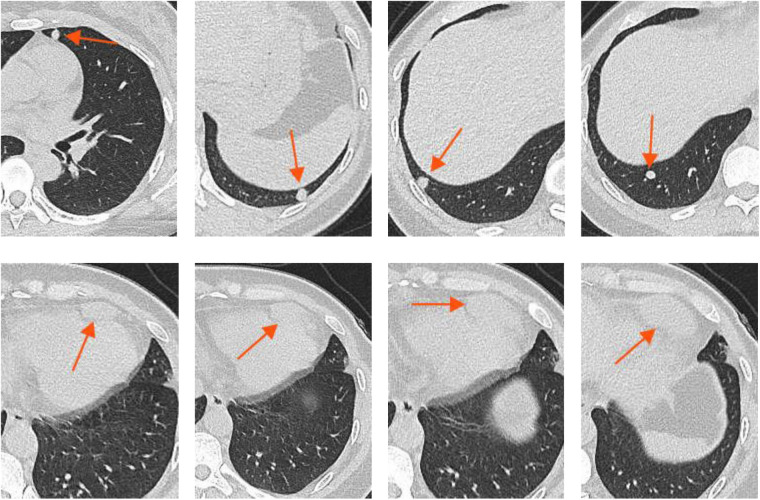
High-resolution non-contrast chest CT.
